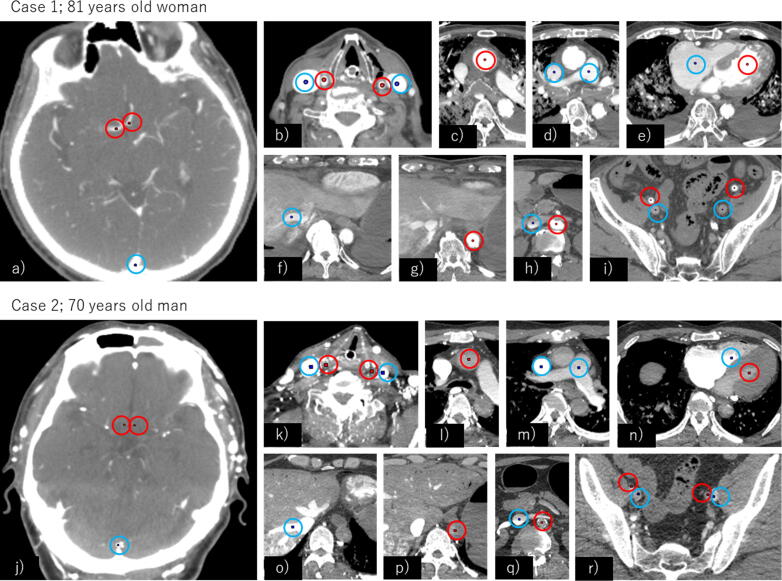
Contrast-enhanced CT of 20 target blood vessels in two representative cases. Red circles indicate arteries, and blue circles indicate veins. Red and blue points denote regions of interest in which CT attenuation value (HU) was measured (a/i/j/r; φ1 mm, b–h/k–q; φ3 mm). a/j. Bilateral anterior cerebral arteries in the circle of Willis and the superior sagittal sinus b/k. Bilateral common carotid arteries and internal jugular veins at the level of thyroid cartilage c/l. Ascending aorta at the level of tracheal bifurcation d/m. Pulmonary artery trunk and superior vena cava at the level of pulmonary artery bifurcation e/n. Left and right ventricles f/o. Hepatic vein at the junction with the inferior vena cava g/p. Descending aorta at the level of the diaphragm h/q. Descending aorta and inferior vena cava at the level of the left renal vein i/r. Bilateral external iliac arteries and veins immediately proximal to the sheath tips.
